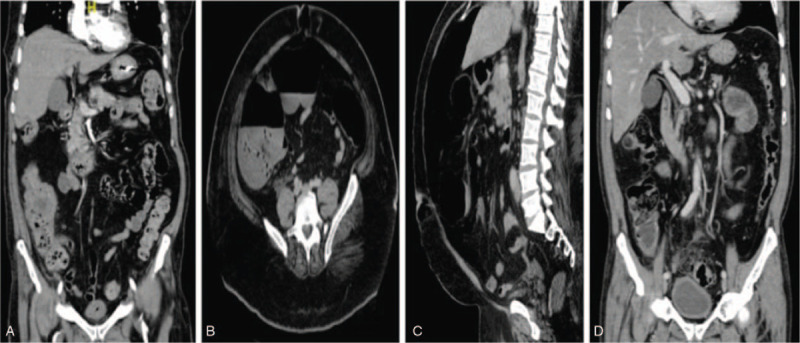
The contrast enhanced computed tomography (CT) of the abdomen of the 3 cases. (A): CT scan abdomen of the 1st case (Coronal image) showing a well-defined rounded heterogeneous enhanced soft tissue mass lesion within the posterior wall of the cecum measuring (3.1 × 3.2 cm) in anteroposterior and transverse diameter associated with discontinuous enhancement of posterior cecum wall and extra-luminal air foci suggestive of complicated perforated sealed cecum mass. This is in addition to adjacent fat stranding with free fluid as well as enhancement of peritoneal reflection suggestive of peritonitis. (B &C): CT scan abdomen of the 2nd case (Axial & Coronal images). (2B): Axial image showing moderate to severe pneumoperitoneum with air seen more tracking along the ascending colon suggestive of a wall defect in the anterior aspect of the cecum. (2C): Coronal image showing a second defect in the stomach wall. (D): CT scan abdomen of the 3rd case (Coronal image) showing severe sigmoid diverticulosis with circumferential bowel wall thickening compatible with acute diverticulitis, small amount of free air compatible with bowel perforation likely arising from the sigmoid colon and a well-defined 3.3 × 1.5 cm abscess collection adjacent to the sigmoid colon.
